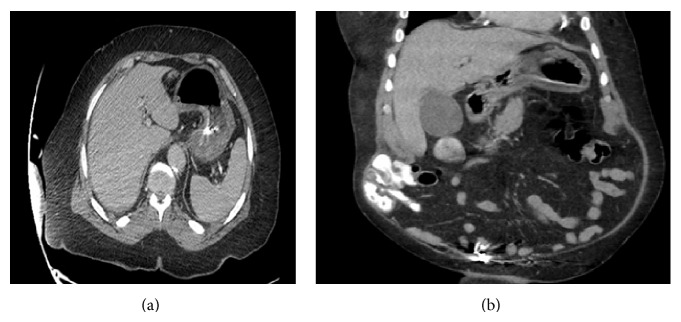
Contrast enhanced CT scan of the abdomen (48 hours after presentation) showing resolution of gas in the wall of the stomach, portal veins, and spleen.
